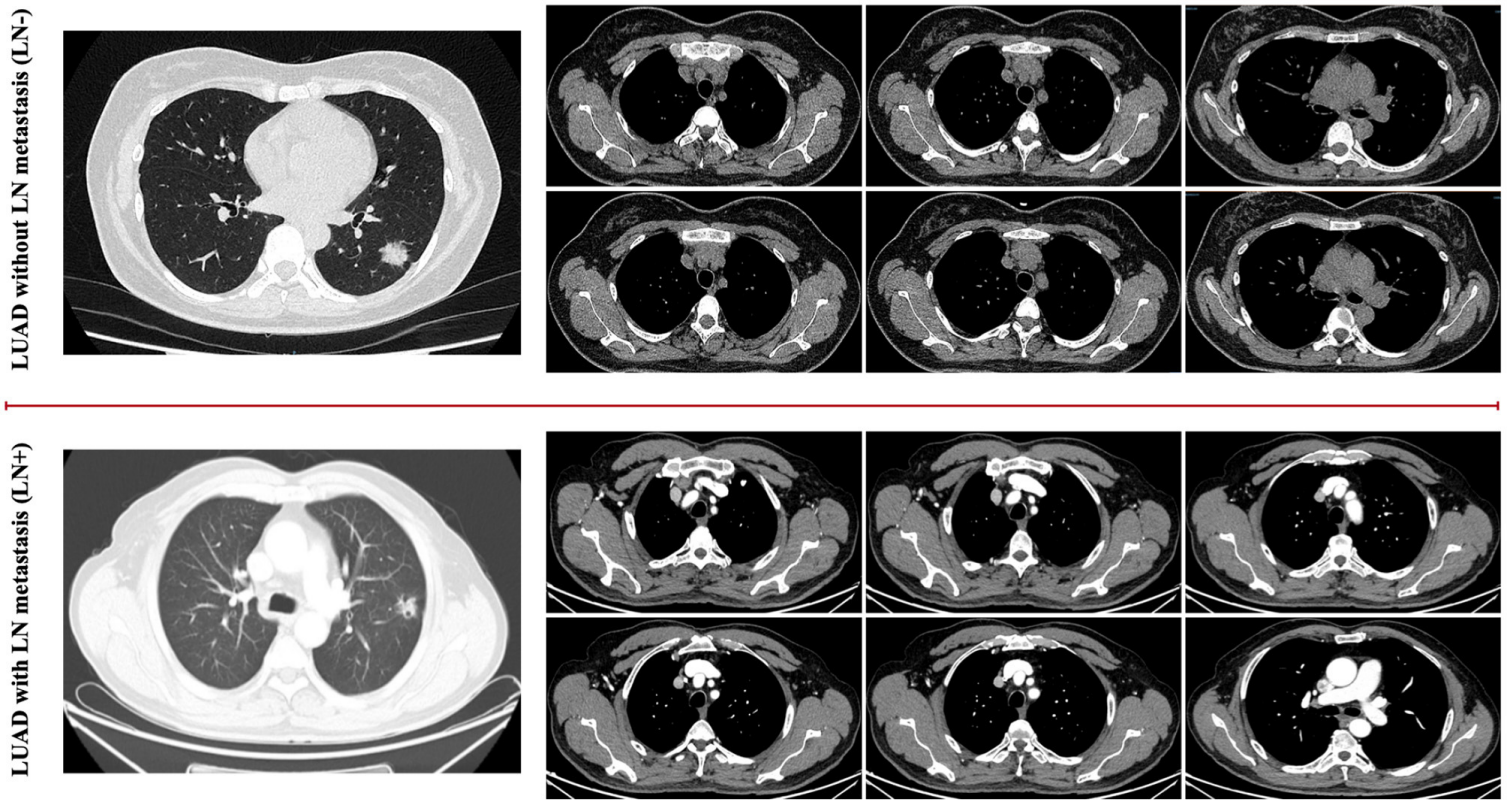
It is difficult for humans to identify the difference between the LUAD with LN metastasis cases as well as the LUAD without metastasis cases based on the general visualized CT images, as shown in the examples for comparison.
